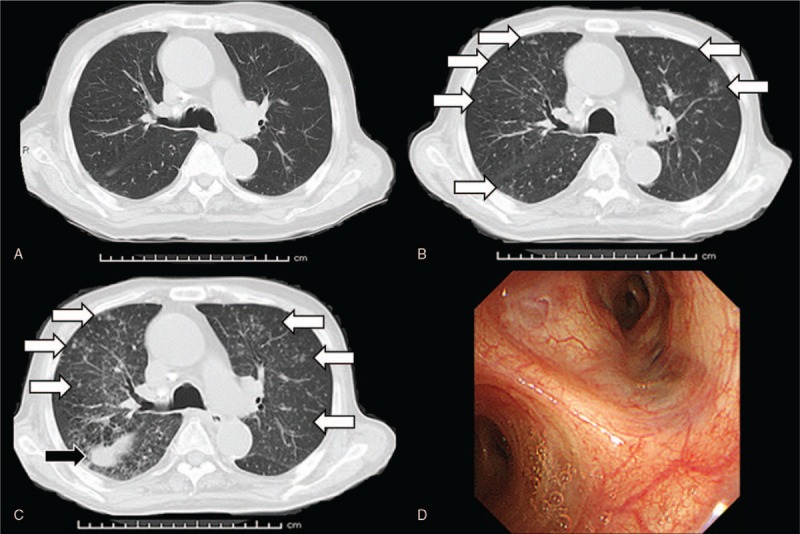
Compared with the findings of chest computed tomography before cabazitaxel treatment (A), small nodules distributed around the bilateral lungs (white arrows) emerged 15 days after the third course of cabazitaxel therapy (B). Findings of diffusely distributed small nodules worsened (white arrows) and pleural effusion (black arrow) was newly observed 23 days after the third course of cabazitaxel therapy (C). Bronchoscopy of the respiratory system did not yield any significant findings (D).
